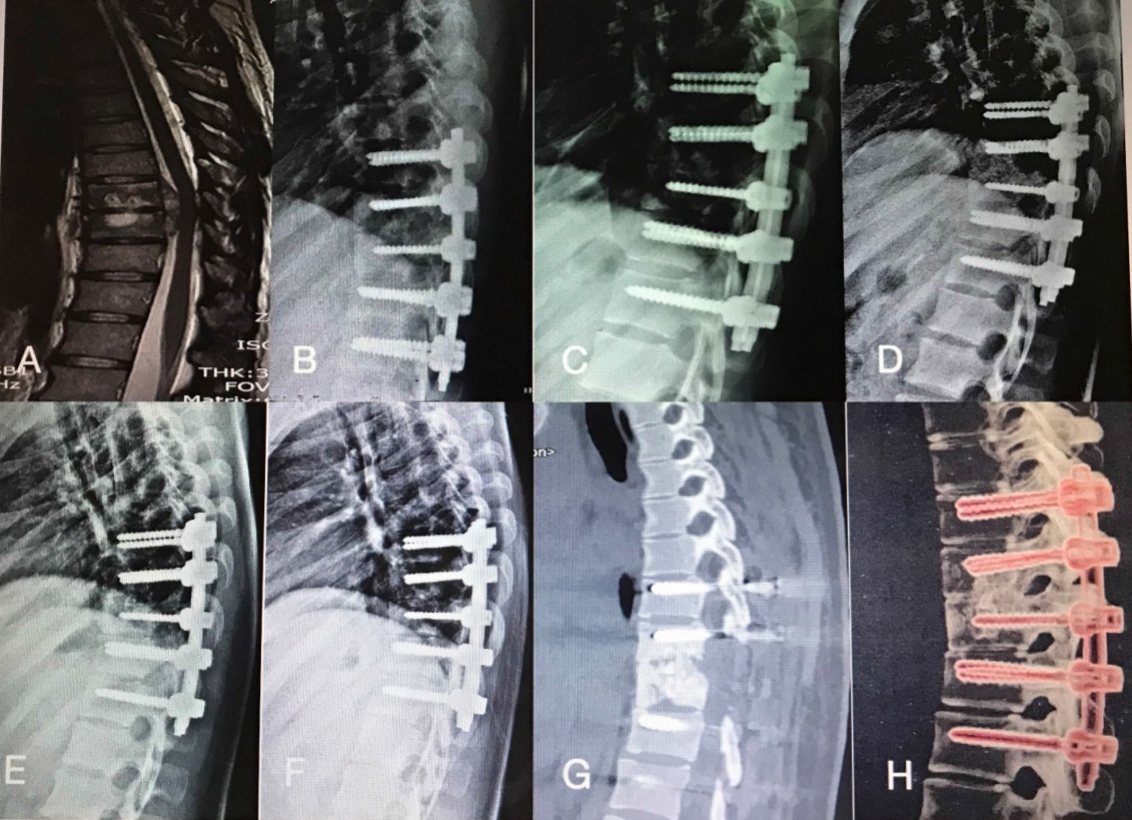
A 45-year-old man with thoracic spinal tuberculosis (T9-10)underwent single-segment posterior debridement and decompression combined with internal fixation. (A)Pretreatment MRI showing the destruction of the T9-10vertebrae and concomitant compression of the spinal cord. (B,C,D,E) Immediate, 3-month, 6-month, 1-year, 2-year postoperative X-ray demonstrated good location of bone graft and internal fixation. (F,G,H) At the 36-month follow-up, plain X-ray and CT showed maintenance of the correction and solid fusion.
